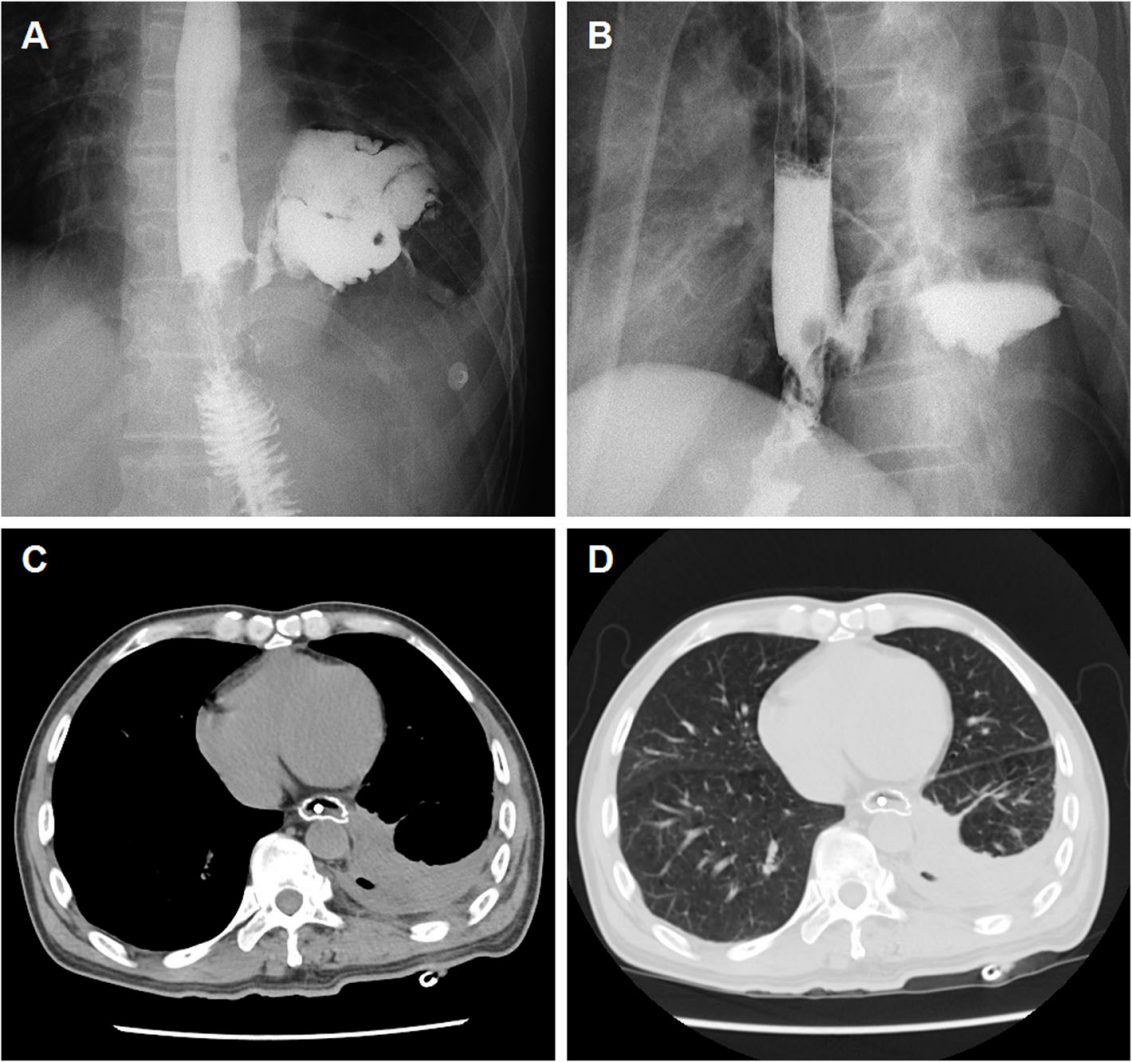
A 59-year-old man with anastomotic leak after esophagojejunostomy (case 24). a-b Esophagography showing an anastomotic leakage in the lower esophagus and irregular abscess in the pleural cavity. c–d Chest CT scan in the mediastinal and lung windows show mediastinal abscess and a small amount of pleural effusion before procedure
